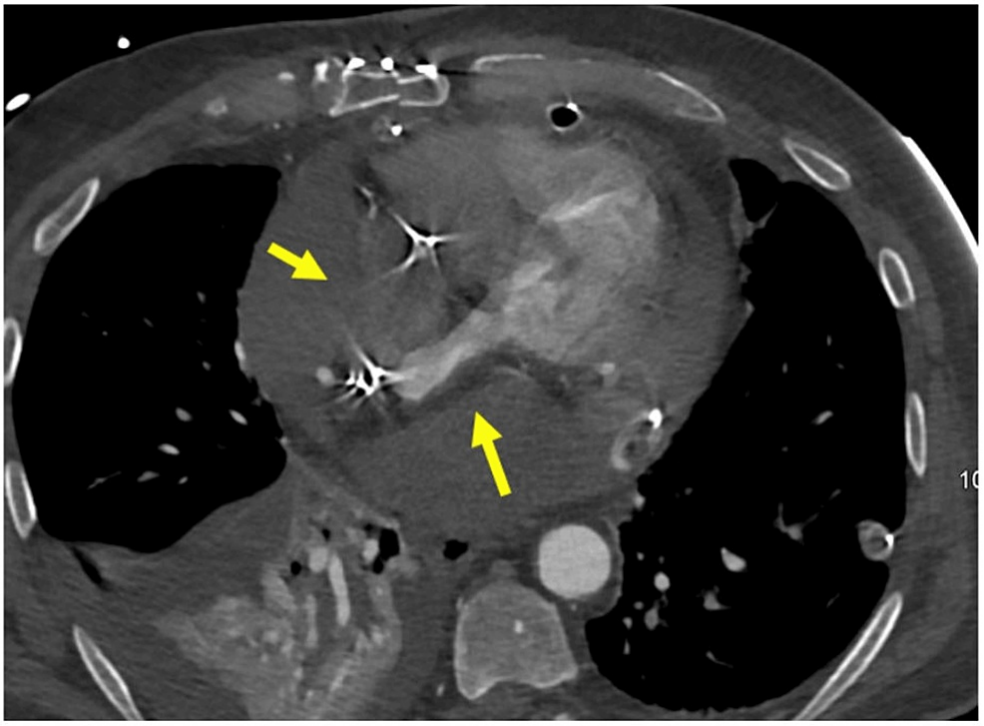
Arterial phase spiral CT angiogram showing acute tamponade with compression on the atria (yellow arrows).
